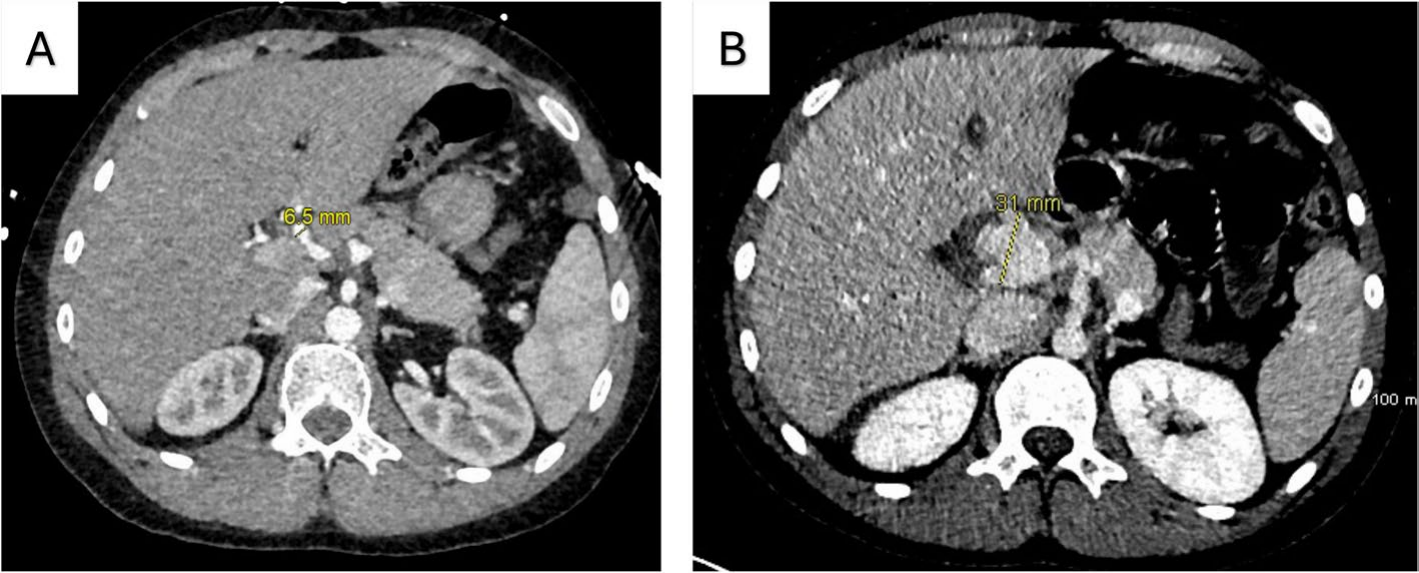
Comparison of pre-laparotomy (A) and post-laparotomy CT scans (B). A Pre-laparotomy CT shows the common HA at 6.5 mm. B Common HA dilated to 31 mm in 5 days
